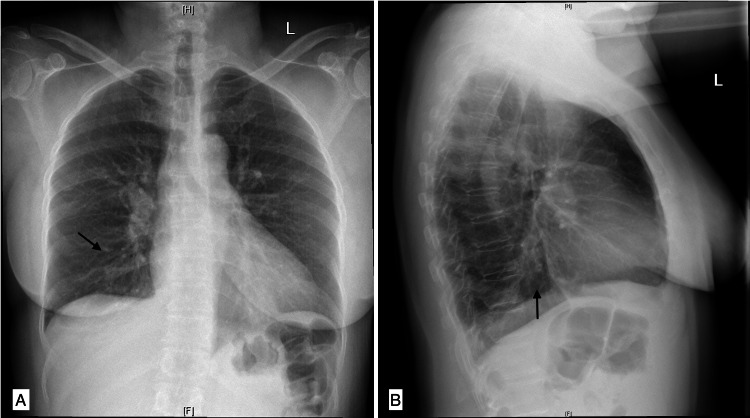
Initial chest radiography A) posterior-anterior view; B) lateral view Arrows indicate subtle lower lobe opacities.
